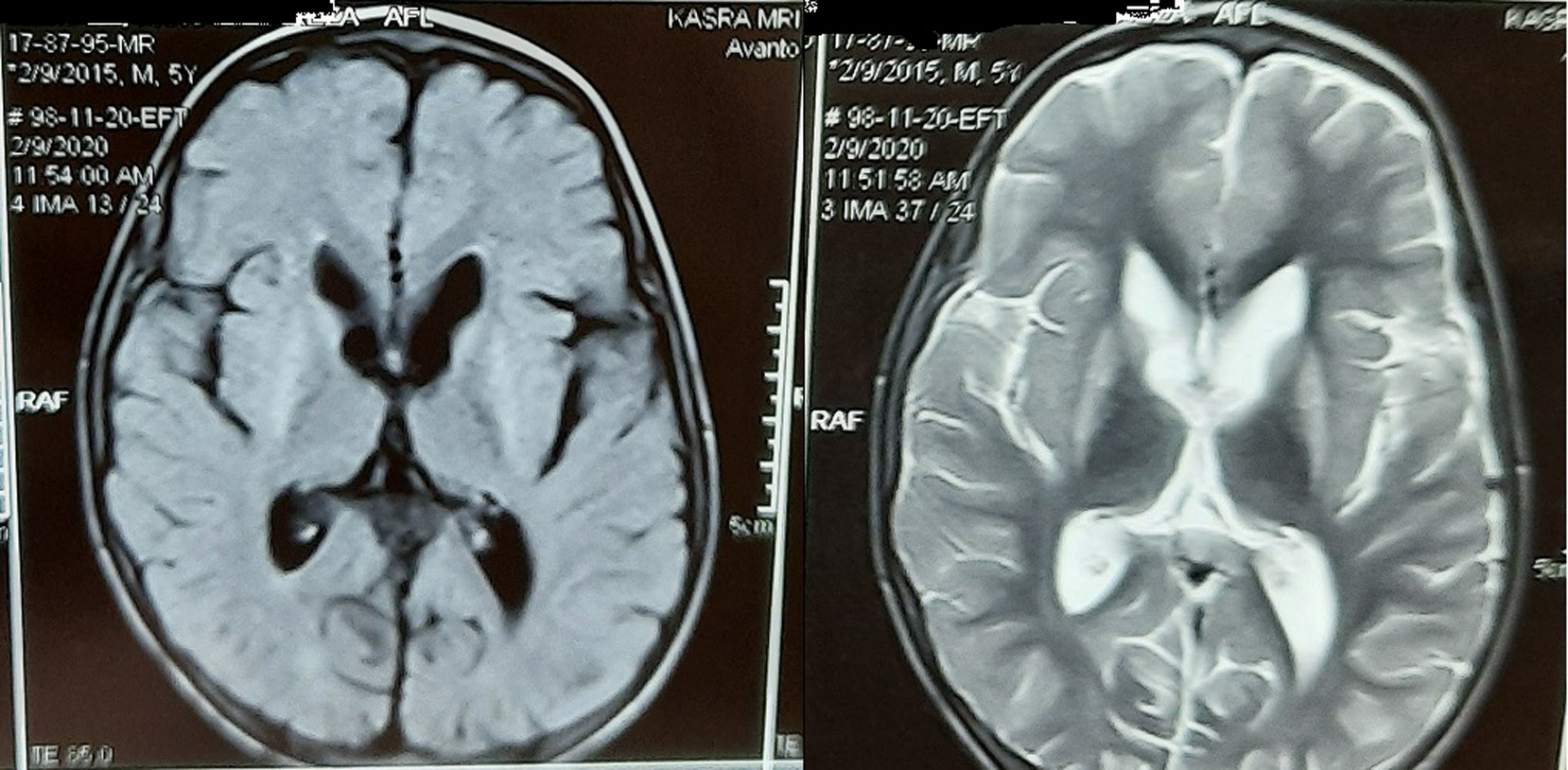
Axial FLAIR MR image (left) and axial T2W image (right) show abnormal high signal changes in bilateral caudate and putamen (striatum)
